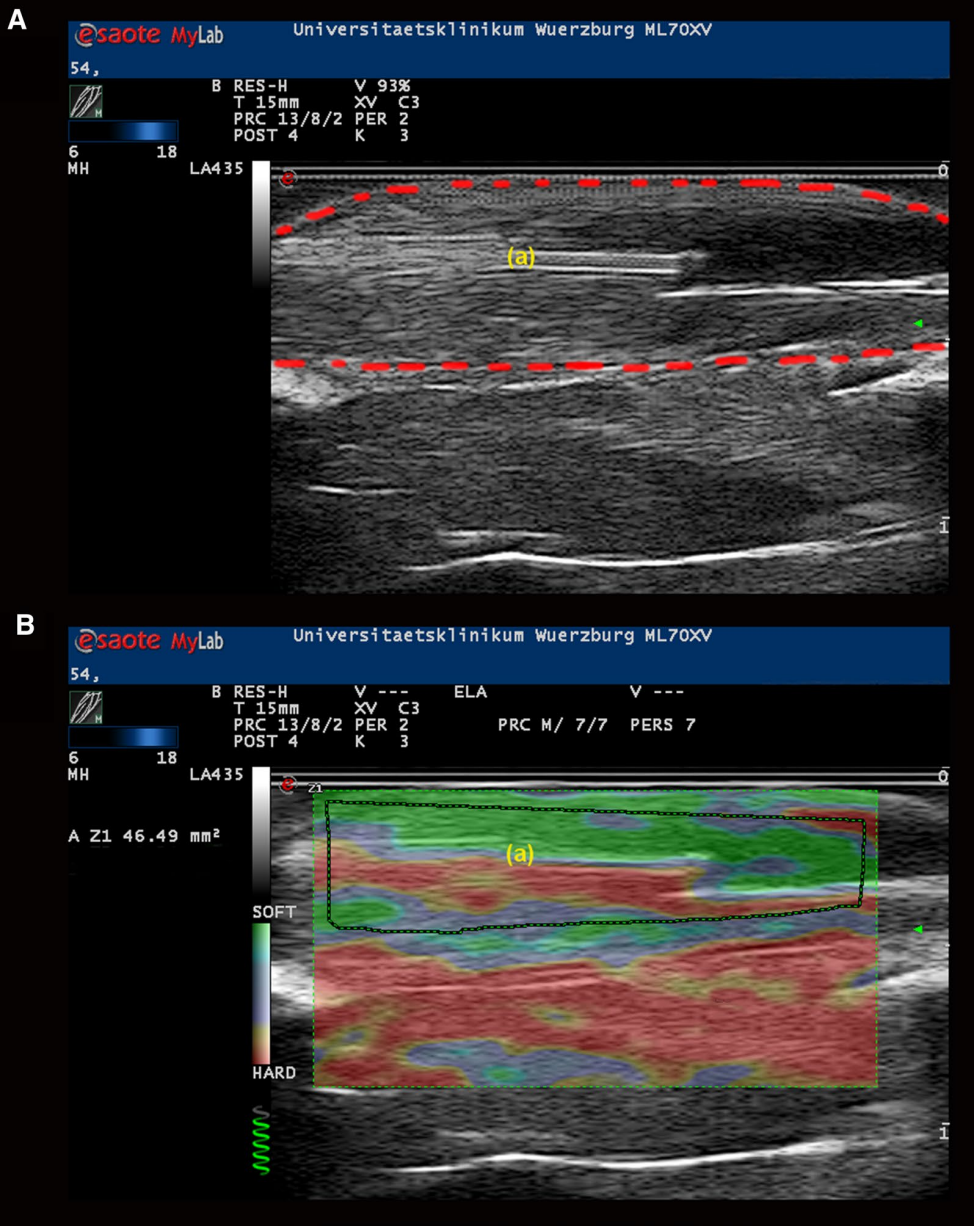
A B-mode ultrasound image (exemplarily) of anterior tibial muscle (red dotted line) with microdialysis probe inserted (a). B Superimposed color-coded strain elastography image with selected measuring field in the anterior tibial muscle (dotted line) around the microdialysis probe (a)
